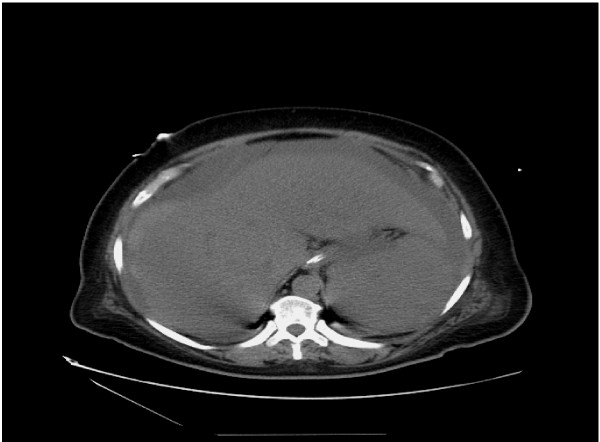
Computed tomography scan of the abdomen without contrast after the liver biopsy showed acute hemorrhage (arrows). The unenhanced liver is normal in size and attenuation.
